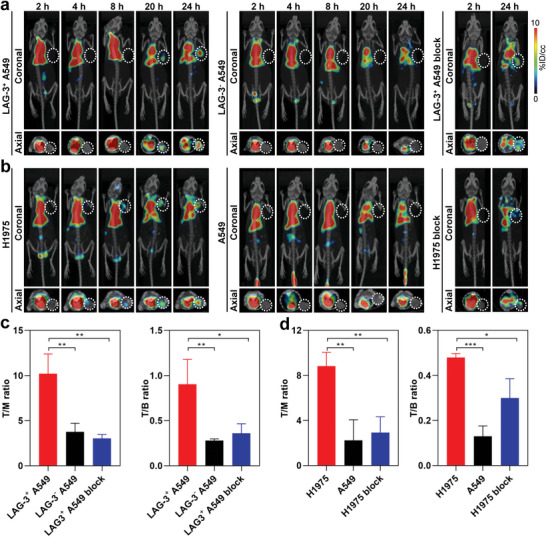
SPECT/CT imaging of ^99m^Tc‐HYNIC‐αLAG‐3 and ^99m^Tc‐HYNIC‐αPD‐L1 in lung cancer models. Representative coronal and axial SPECT/CT images of ^99m^Tc‐HYNIC‐αLAG‐3 a) and ^99m^Tc‐HYNIC‐αPD‐L1 b). T/M and T/B ratio of ^99m^Tc‐HYNIC‐αLAG‐3 c) and ^99m^Tc‐HYNIC‐αPD‐L1 d). White dotted circles indicate tumors. For insets c and d, data are presented as mean ± SD, *n* = 3. ^*^
